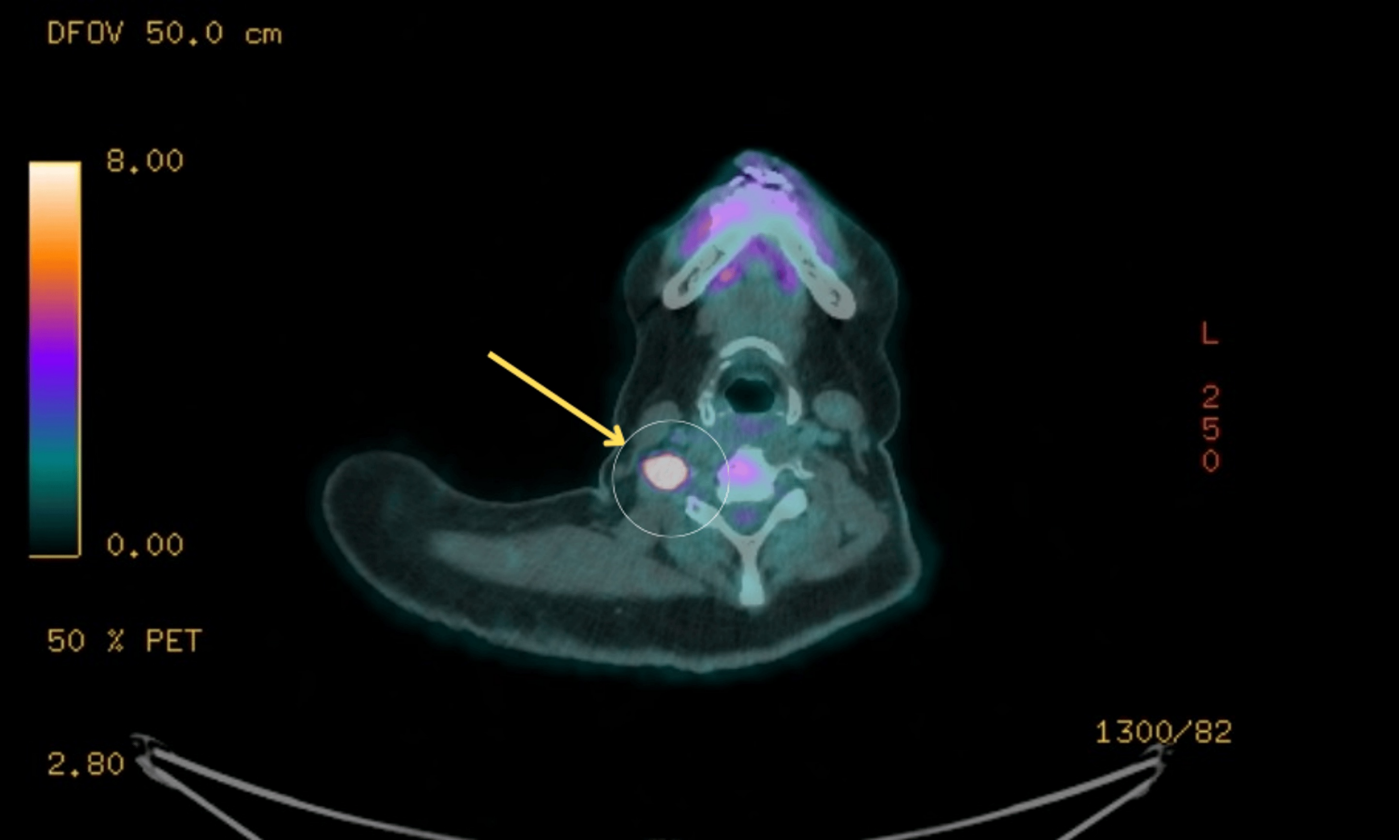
FDG-avid lesion at the right C5 nerve root on PET-CT Axial PET-CT image demonstrating intense fluorodeoxyglucose (FDG) uptake at the right C5 nerve root (yellow arrows). This hypermetabolic focus is suggestive of active lymphomatous infiltration of the cervical nerve root, supporting a diagnosis of central nervous system lymphoma.
